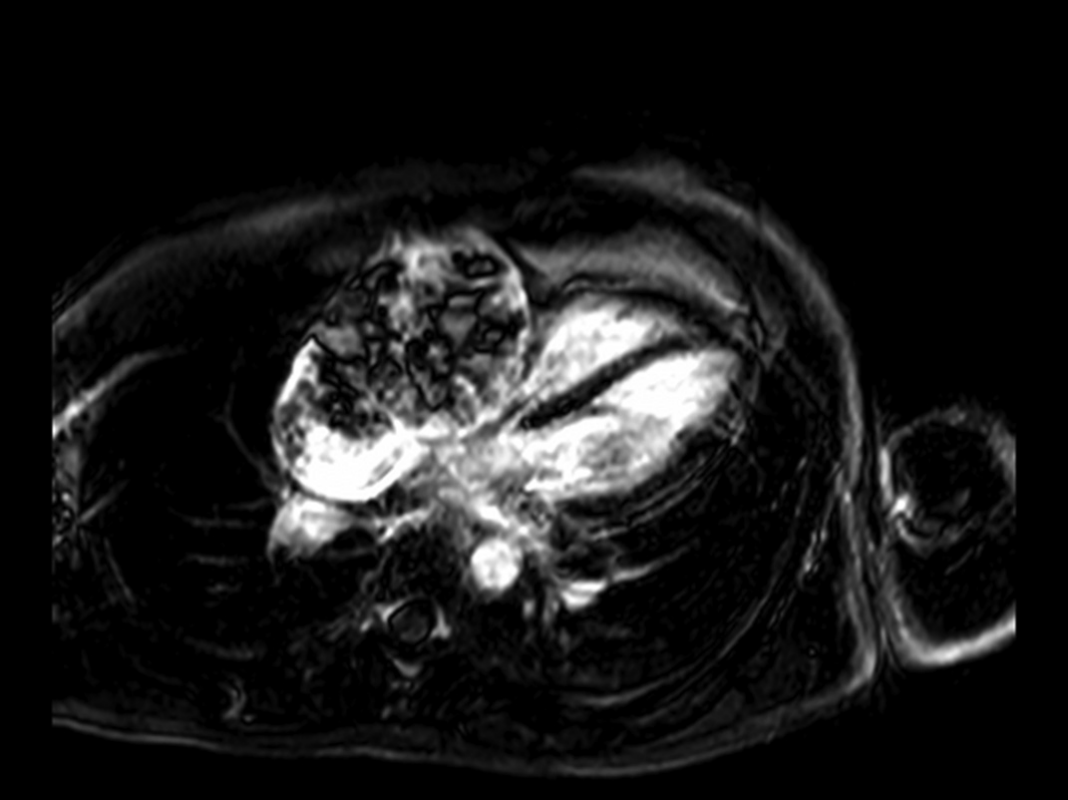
Late Gadolinium Enhanced Cardiac Magnetic Resonance Image of the Right Atrial Mass
